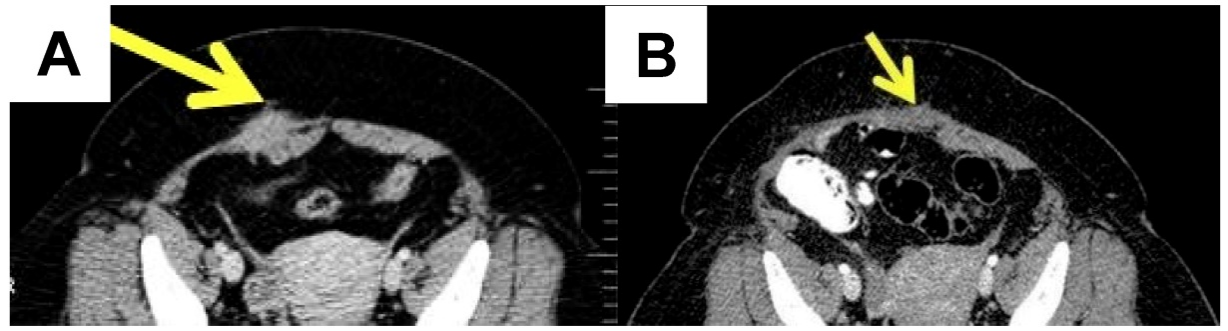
A case of a 44-year-old female diagnosed with abdominal wall endometriosis 14 years after a caesarean section. She had chronic pain unrelated to the menstrual cycle. A. Preoperative aspect: computed tomography showing a poorly defined tumour of 3.9 cm at the abdominal wall, with a heterogeneous aspect. B. Post-operative aspect by computed tomography.
